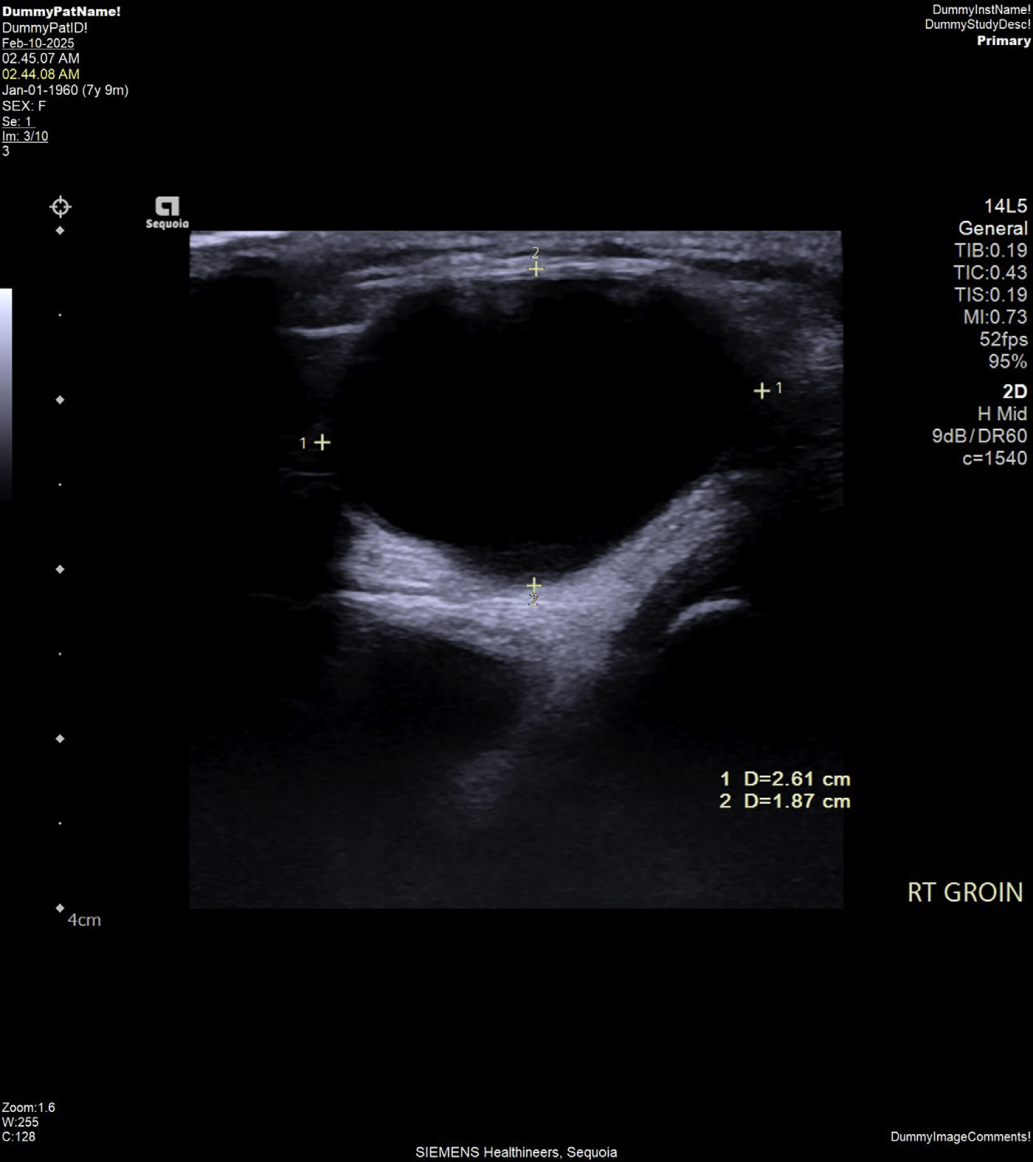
Gray-scale ultrasound image of the right groin showing an elliptical anechoic cystic lesion positioned laterally to the epigastric vessels measuring 2.6 × 1.9 cm. No herniation of pelvic organs or bowel loops was seen.
